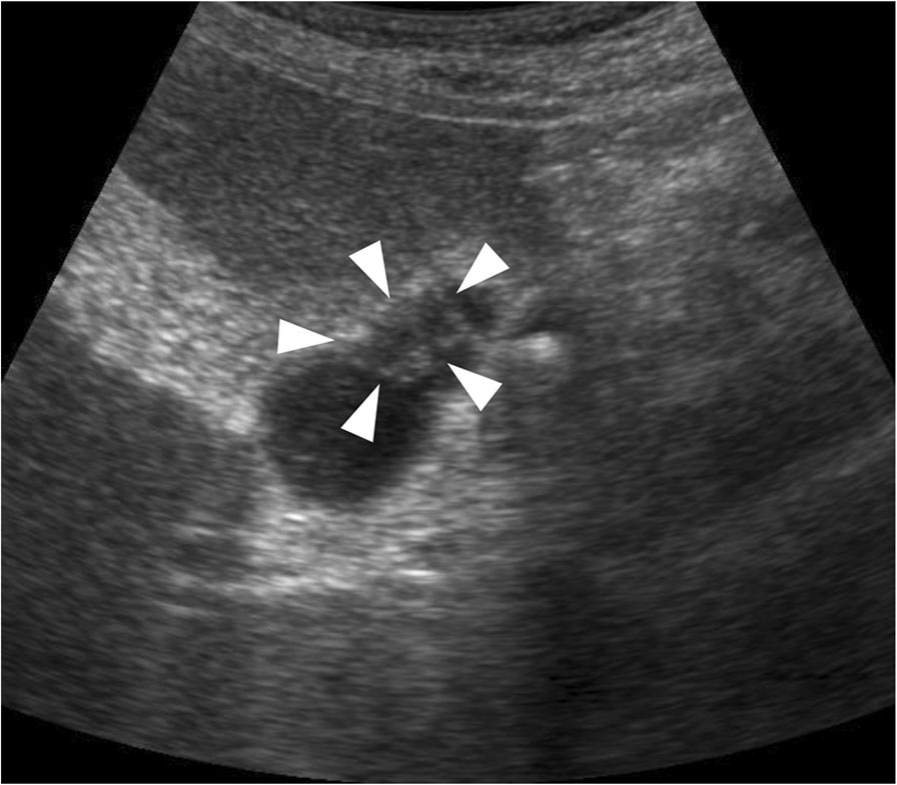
Ultrasonography image shows a 0.8-cm low echoic nodule (arrowheads) in the neck of the gallbladder, which was preoperatively suspected to be a Rokitansky-Aschoff sinuses
